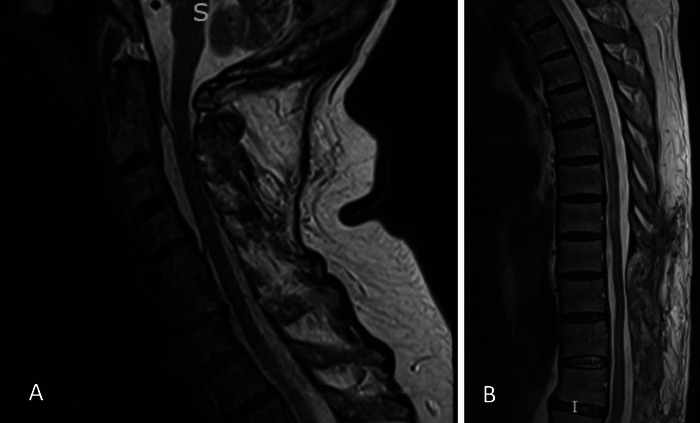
Postoperative sagittal T2 magnetic resonance imaging sequence of (A) the cervical spine and (B) the thoracolumbar spine shows resolution of epidural abscess.
